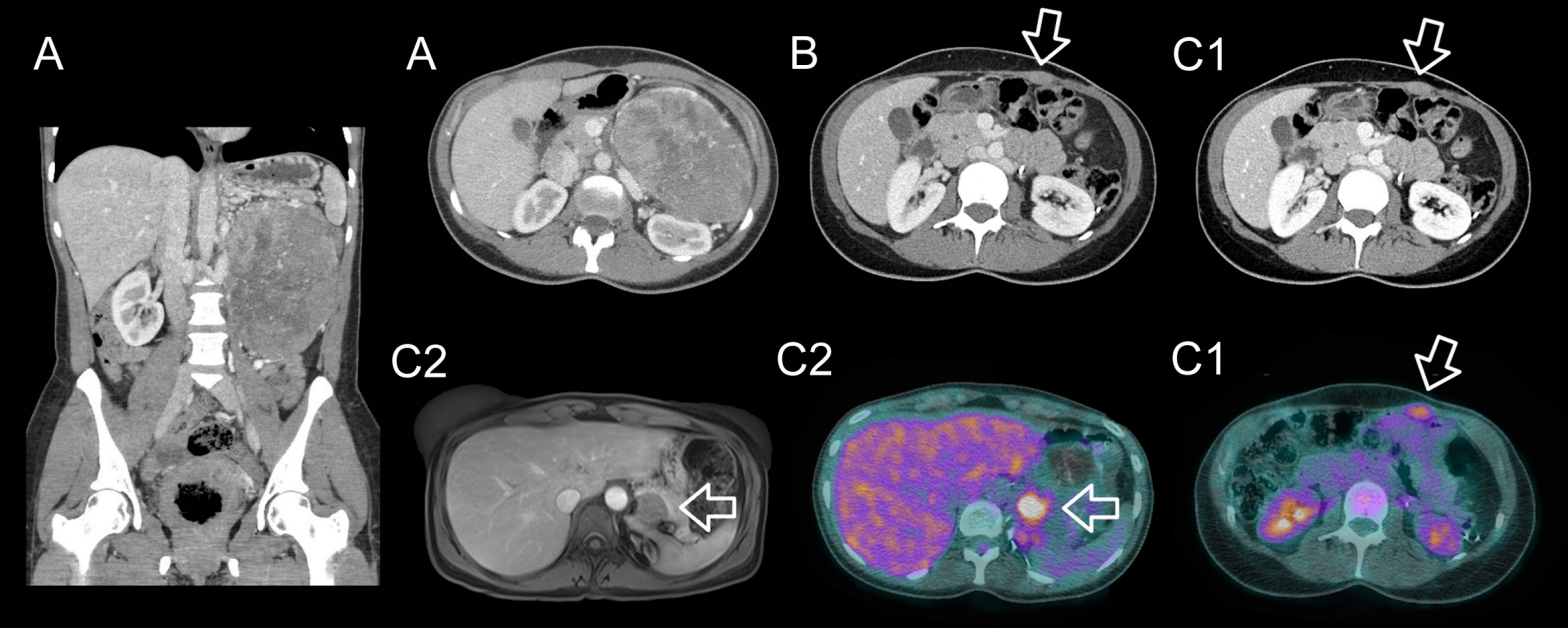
(A) A 15 cm, heterogenous, retroperitoneal mass is shown on coronal (left panel) and axial (right panel) abdominal CT imaging with i.v. contrast. (B) The first abdominal wall recurrence, 2 years following initial surgery: a 3 cm mass is seen in the left anterior abdominal wall on CT (arrow). (C) Second recurrence, 3 years after surgery. (C1) A 2.1 × 1.3 cm left anterior abdominal wall lesion is seen respectively on CT (upper panel) and FDG-PET (lower panel) (arrow). (C2) A 2.2 × 1.5 cm retroperitoneal recurrence is shown, on the superior pole of the left kidney, behind the splenic vein at the initial tumor bed, respectively on MRI (left panel) and FDG-PET (right panel) (arrow).
